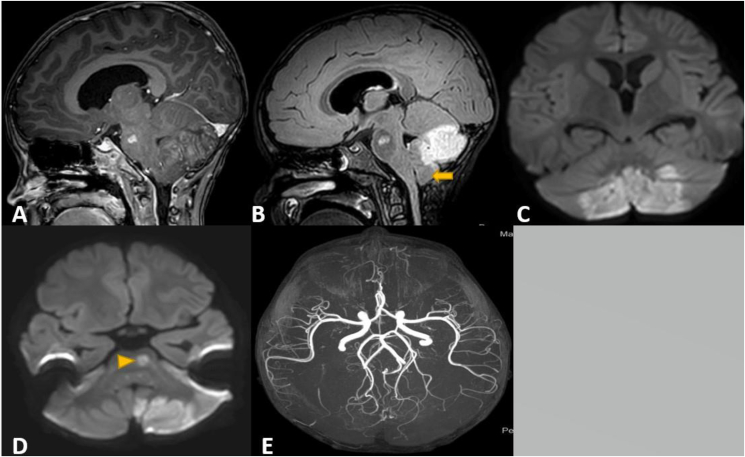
Magnetic resonance imaging of the brain (MRI). A: Sagittal view with contrast showing cerebellar gyri and pontine enhancement with mild tonsillar herniation, B: Flair sequence showing cerebellar edema, mild hydrocephalus and 3 mm tonsillar herniation (arrow), C–D: Diffusion-Weighted view showing avid restriction involving pons (arrowhead) and the medial inferior aspect of both cerebellar hemispheres confined to the territory of the medial branches of the posterior inferior cerebellar arteries, E: Magnetic resonance angiography (MRA) sequence shows no evidence of arterial stenosis.
